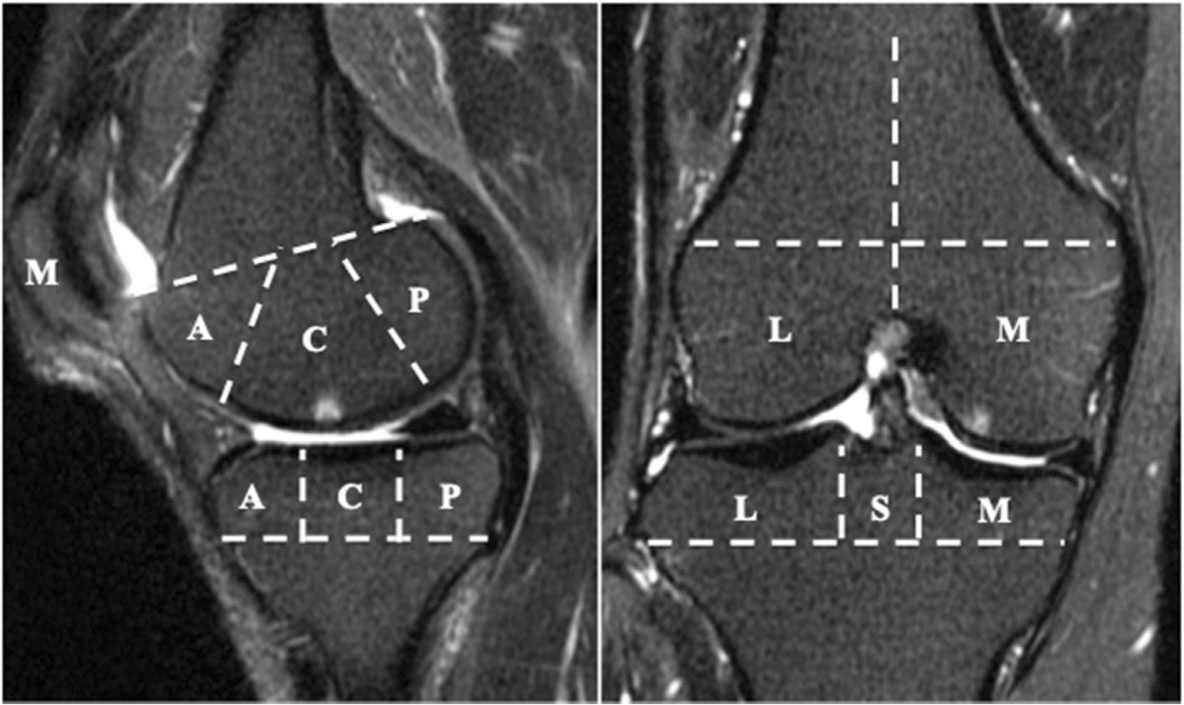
Regional subdivision of the articular surfaces for the assessment of bone marrow lesions (BMLs). BMLs were assessed by the Whole-Organ MRI Scoring (WORMS) method. The patella, femur, and tibia were divided into medial (M) and lateral (L) regions. Region S represents the portion of the tibia beneath the tibial spines. The femoral and tibial surfaces were further subdivided into anterior (A), central (C), and posterior (P) regions
